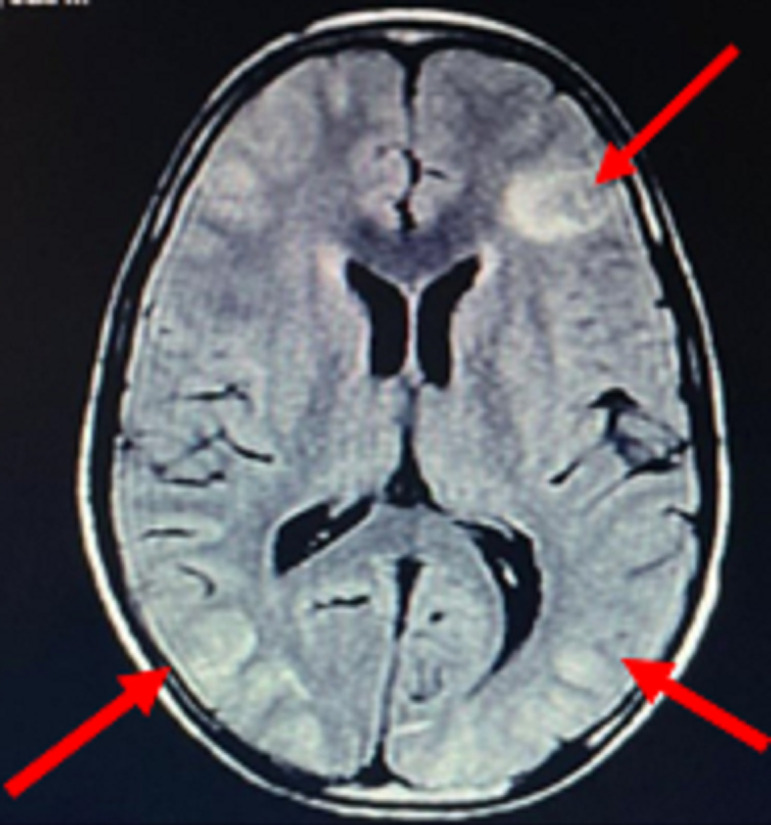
magnetic resonance imaging of brain showing posterior reversible encephalopathy, arrows showing oedematous and hyperintense gyri in bilateral parieto-fronto-occipital region on T2/flair suggestive of posterior reversible encephalopathy syndrome
